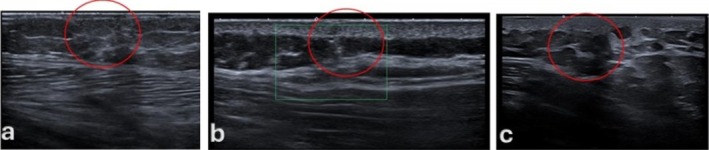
Representative ultra–high‐frequency ultrasound (UHFUS; 48–70 MHz) B‐mode images showing scar‐related dermal alterations across different examinations. The red circles highlight the main area of altered echotexture (typically heterogeneous and/or relatively hypoechoic compared with adjacent dermis), consistent with ongoing scar remodelling.
